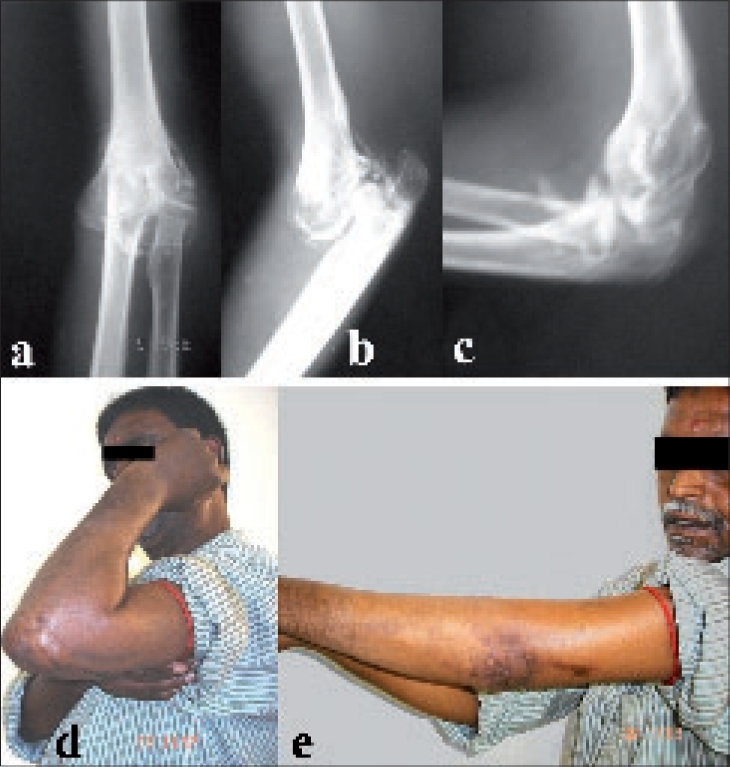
Anteroposterior (a) and lateral radiographs (b) of unreduced posterior dislocation of left elbow with myositis ossificans in an adult male. Postoperative lateral radiograph (c) showing reduced elbow with good range of motion with excised myositis ossificans. Clinical photographs (d and e) showing postoperative range of motion at two years of follow-up
